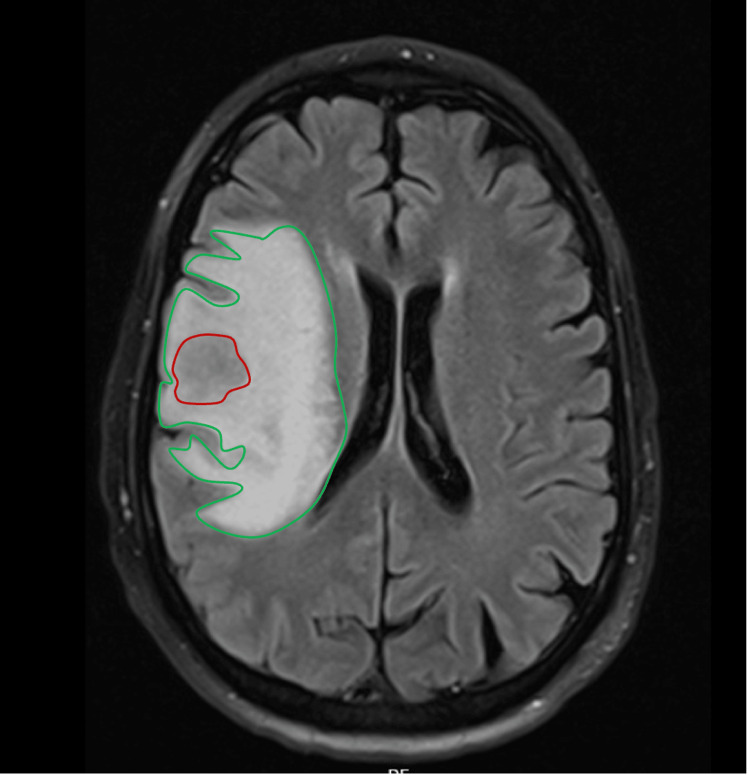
MRI showing a right frontal (outlined in red) mass surrounded by extensive vasogenic edema (outlined in green). Magnetic resonance imaging (MRI) of a right frontal cortico-subcortical mass is shown in a panel of four images: (a) T2 fluid-attenuated inversion recovery (FLAIR) image, showing the lesion (red circle) surrounded by extensive vasogenic edema (green circle).
